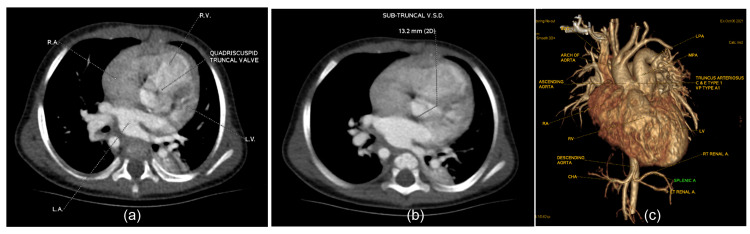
(a) Contrast-enhanced cardiac CT axial image: quadricuspid truncal valve; (b) contrast-enhanced cardiac CT axial image: sub-truncal ventricular septal defect (VSD) of 13.2 mm size; (c) volume rendering (VR) anterior view: truncus arteriosus C & E type 1 and Van Praagh type A1. Right aortic arch giving four branches from anterior to posteriorly—left subclavian artery, left common carotid artery, right common carotid artery, and right subclavian artery. Common hepatic artery arising from superior mesenteric artery and coeliac trunk continuing toward the left side at splenic artery
